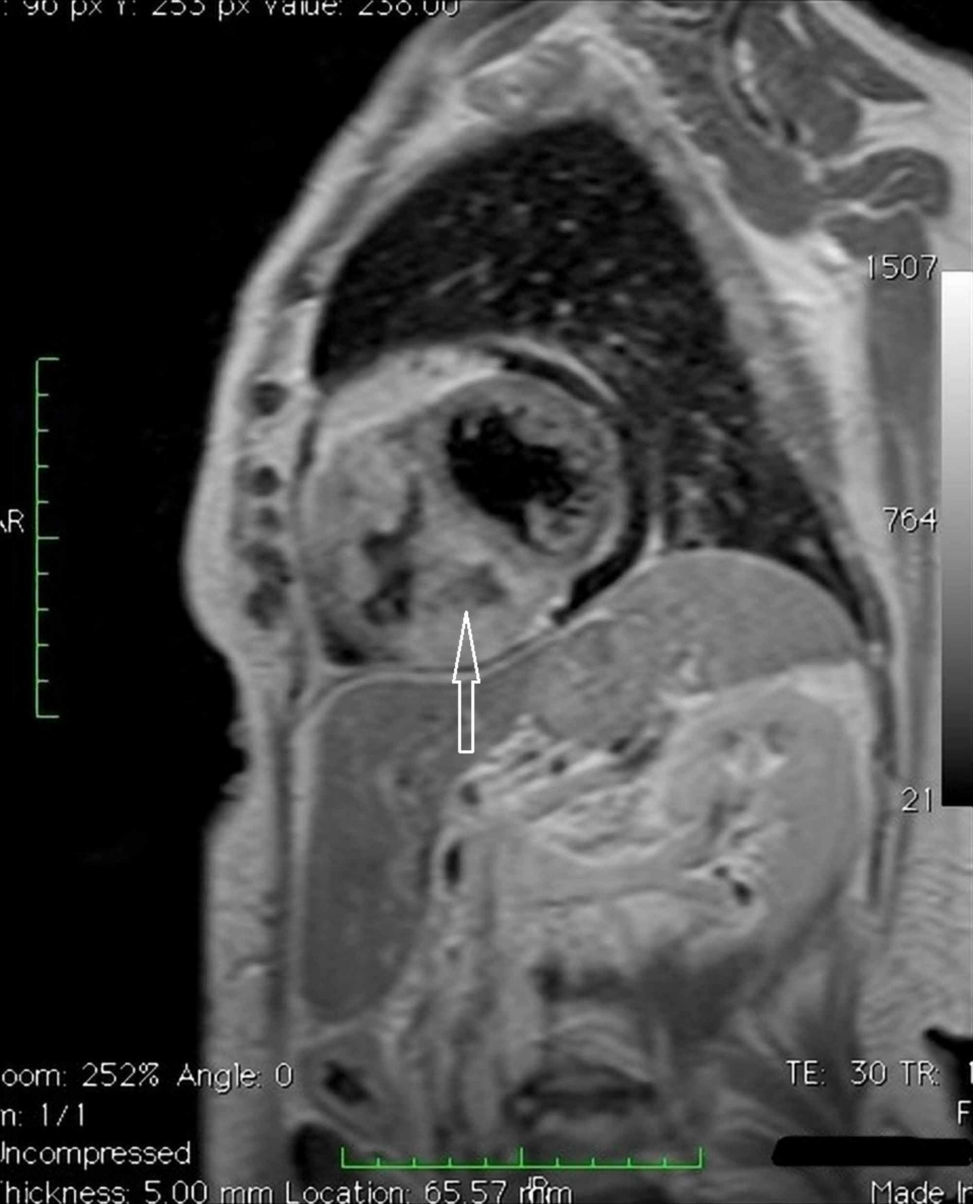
Cardiac magnetic resonance imaging (MRI) TSE_T1 2 chambers cine MRI targeted for the study of the right ventricle.
